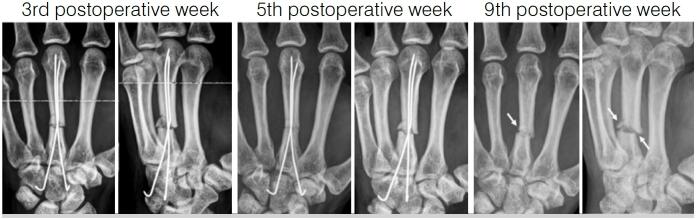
Postoperative follow-up radiographs of a metacarpal fracture. Kirschner wires were removed at the 5th postoperative week after evidence of ultrasonographic consolidation. Radiographic callus formation was observed only starting at the 9th postoperative week.
